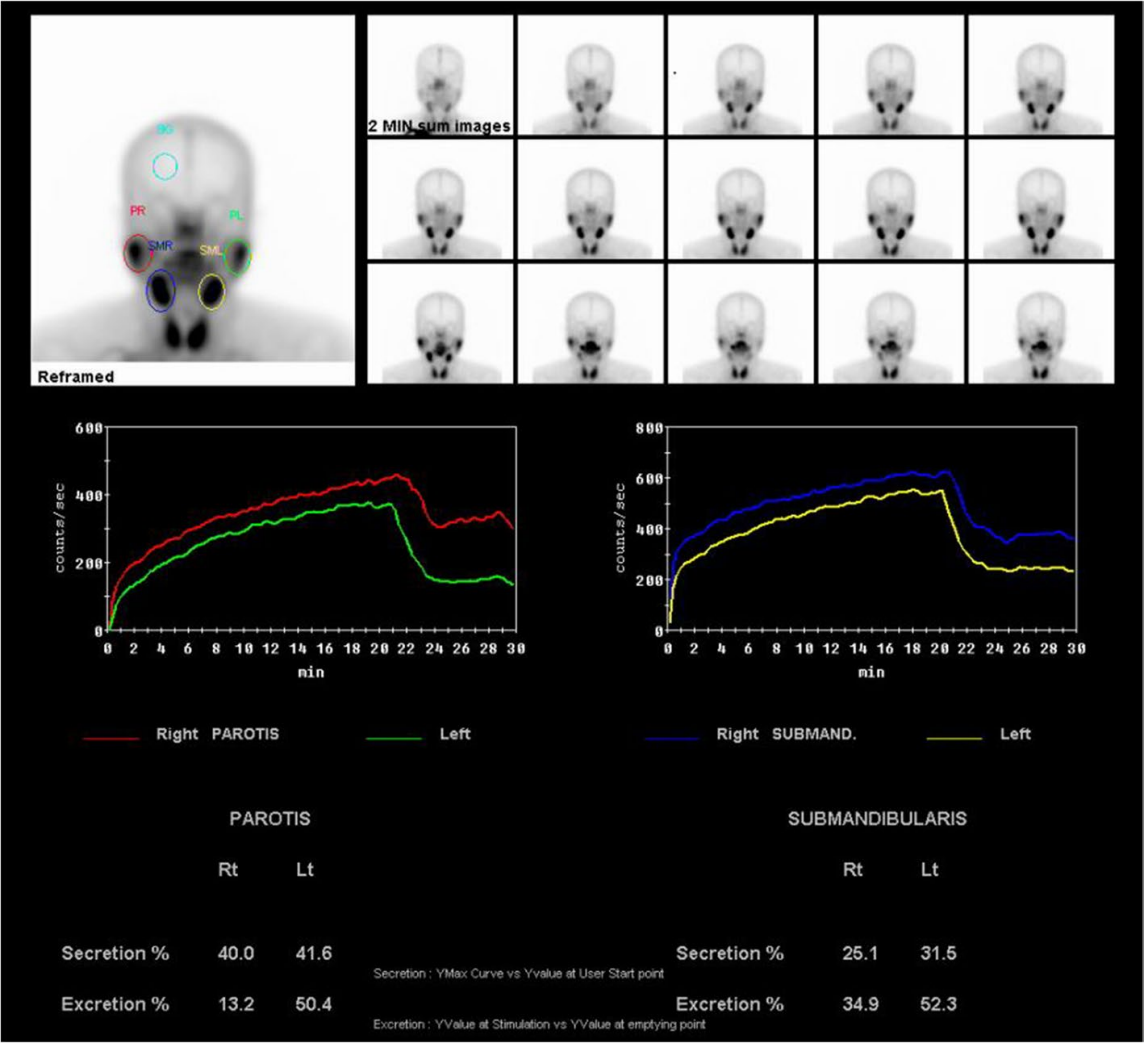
Salivary scintigraphy image with decreased salivary flow
